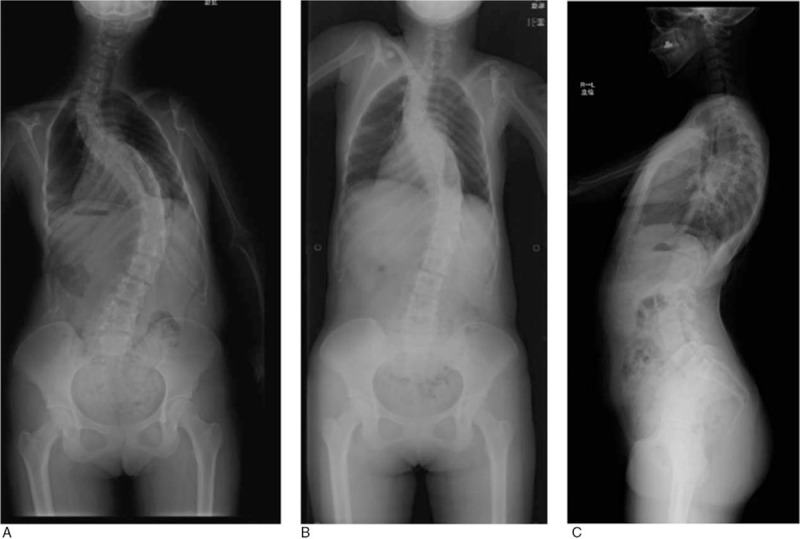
X-ray images. (A) Standing position (P→A); (B) recumbent-traction position (P→A); (C) standing position (sagittal).
